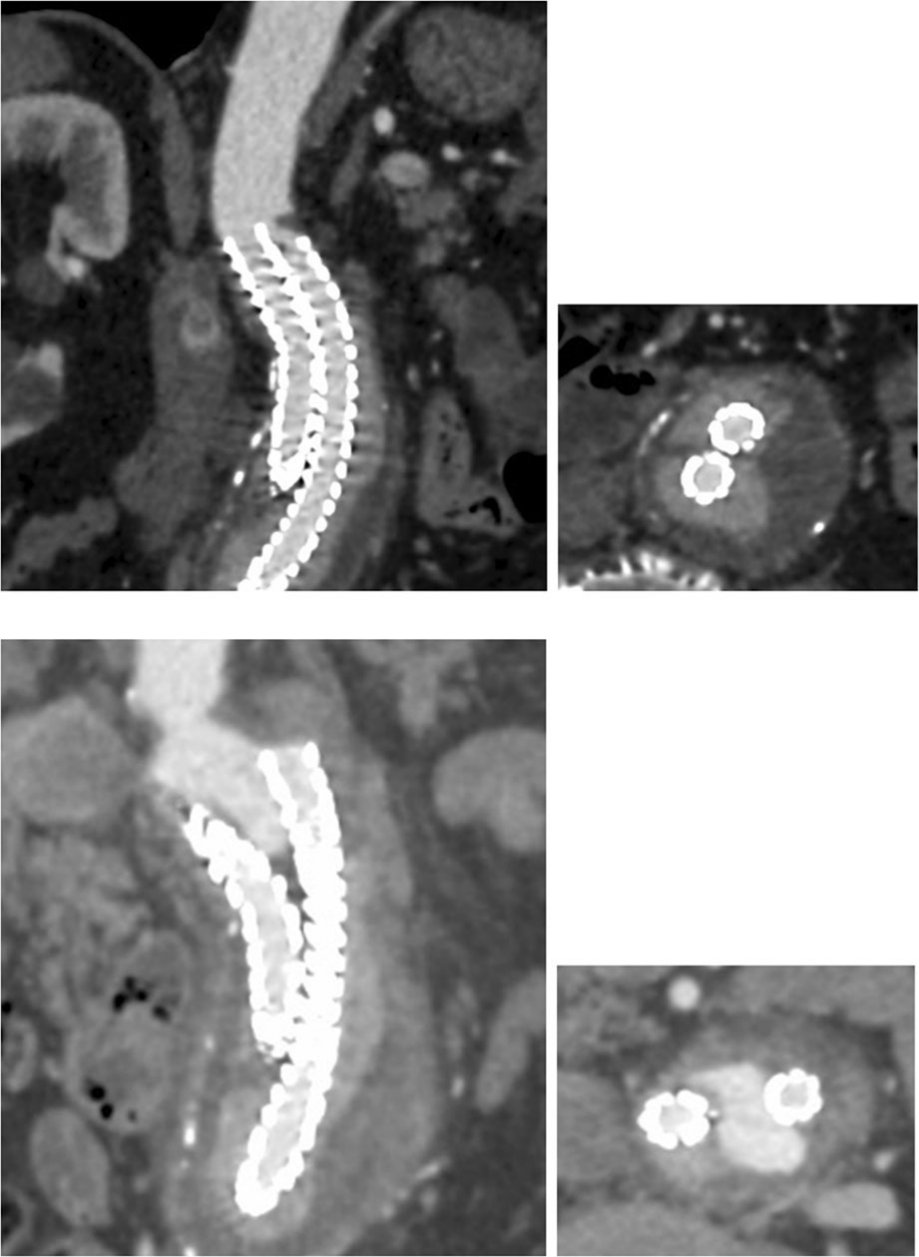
Lateral (on the left) and cross (on the right) views of endobag configuration after implantation (at the top) and after revealing post-operative migration (at the bottom) as obtained with CT scan and Contrast Imaging, as acquired at Sapienza University.
